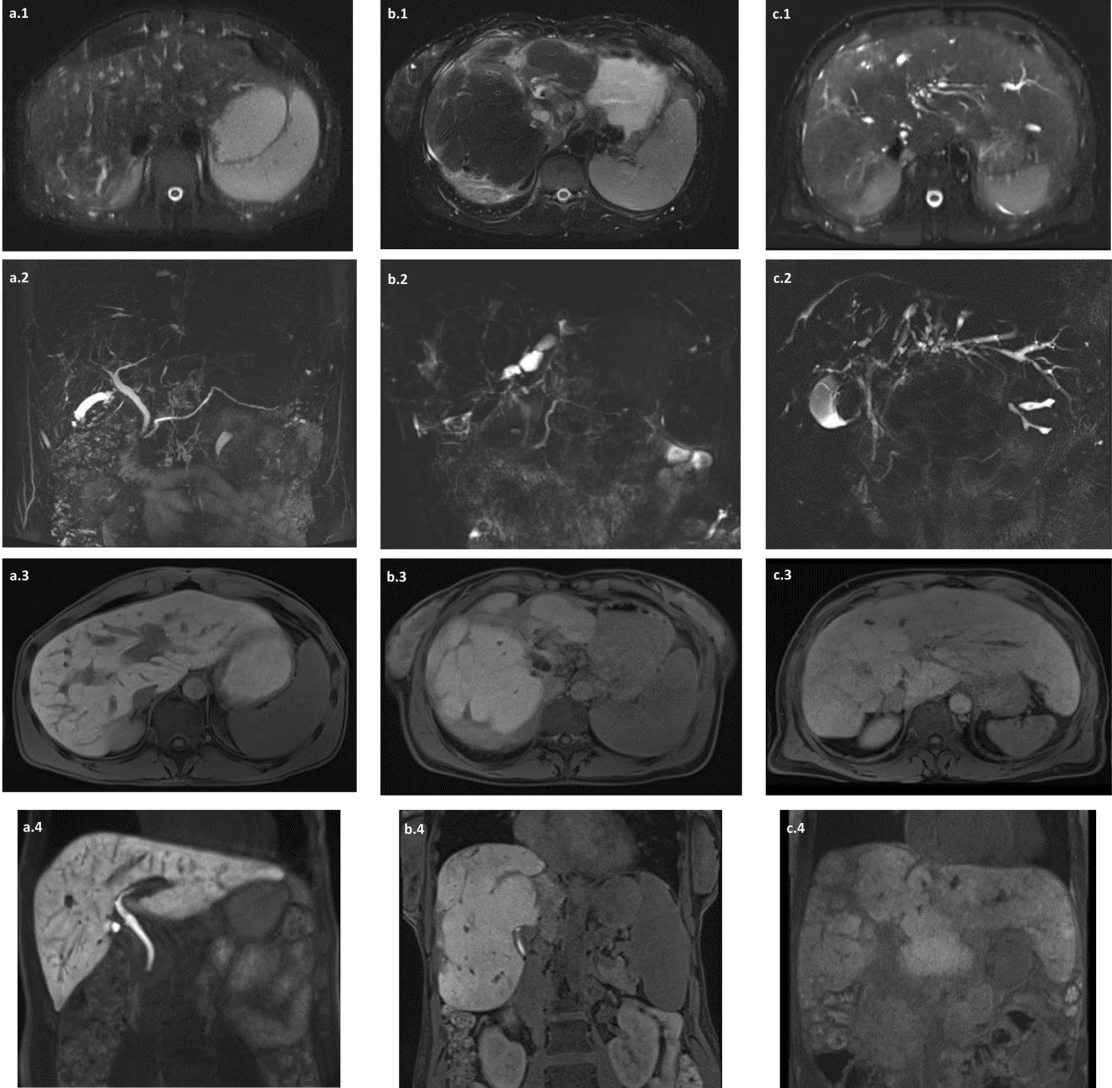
T2-weighted sequence (HASTE) (a1, b1, c1), MRCP (a2, b2, c2), and T1-weighted VIBE sequence 20 min after gadoxetic acid administration (in the HBP) (axial sequence: a3, b3, b3 and coronal sequence: a4, b4, c4) in three patients with different stages of PSC. Patient a: Early PSC changes with peripheral bile duct attenuation and slight T2 hyperintensity in the right posterior liver segment. Gadoxetic acid-enhanced images in the HBP demonstrate homogenous parenchymal enhancement and regular excretion of gadoxetic acid in the CBD. Patient b: Typical advanced PSC changes with focal impairment of liver parenchyma in the form of significant segmental bile duct stenosis, T2 hyperintensity, and atrophy affecting most liver segments apart from segments V and VIII. Gadoxetic acid-enhanced images in the HBP demonstrate heterogeneous parenchyma with absent enhancement in affected segments and absent excretion of gadoxetic acid in the CBD. Patient c: End-stage PSC reflected by cirrhotic liver configuration, multisegment bile duct stenosis and atrophy, as well as absent contrast enhancement and excretion of gadoxetic acid in the CBD in the HBP. *CBD* common bile duct; *HASTE* half-Fourier acquisition single-shot turbo spin-echo; *VIBE* volumetric interpolated breath-hold sequence; *HBP* hepatobiliary phase; *PSC* primary sclerosing cholangitis
